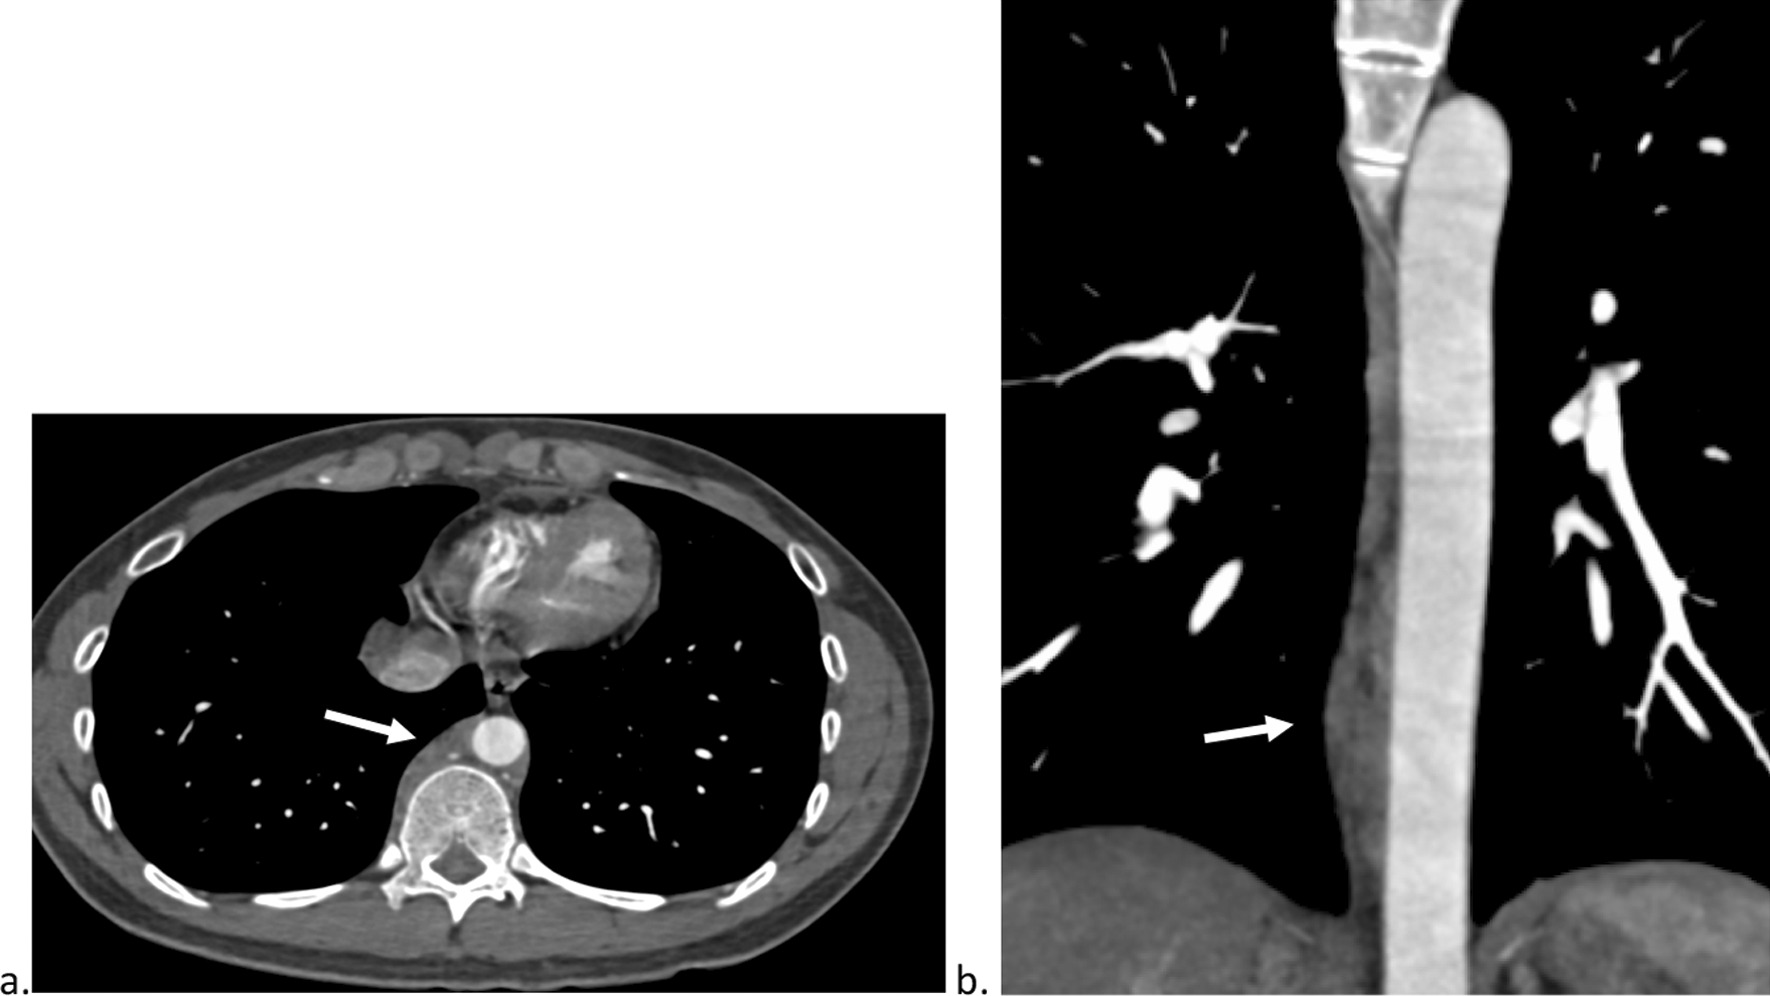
Axial (a) and coronal (b) images from a contrast-enhanced CT of the chest. Soft tissue density adjacent to the aorta and vertebral body (arrow) is evident
